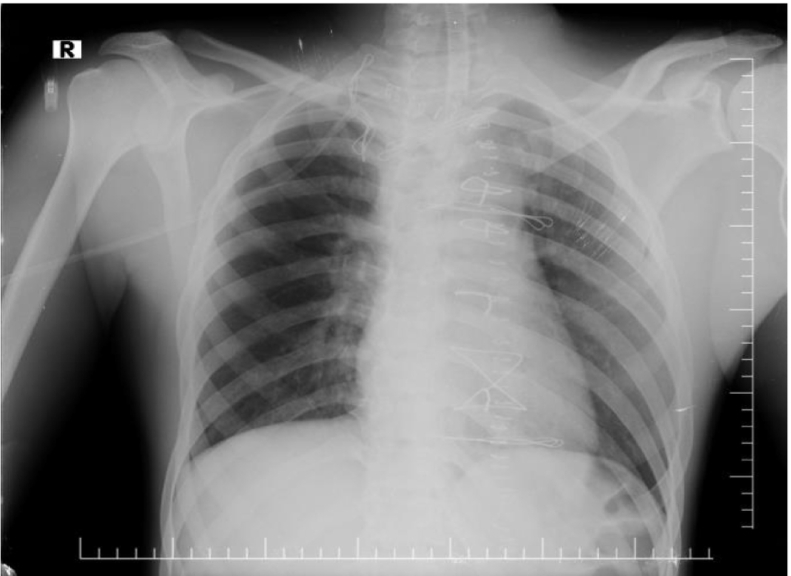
Normal post-op chest x-ray.
